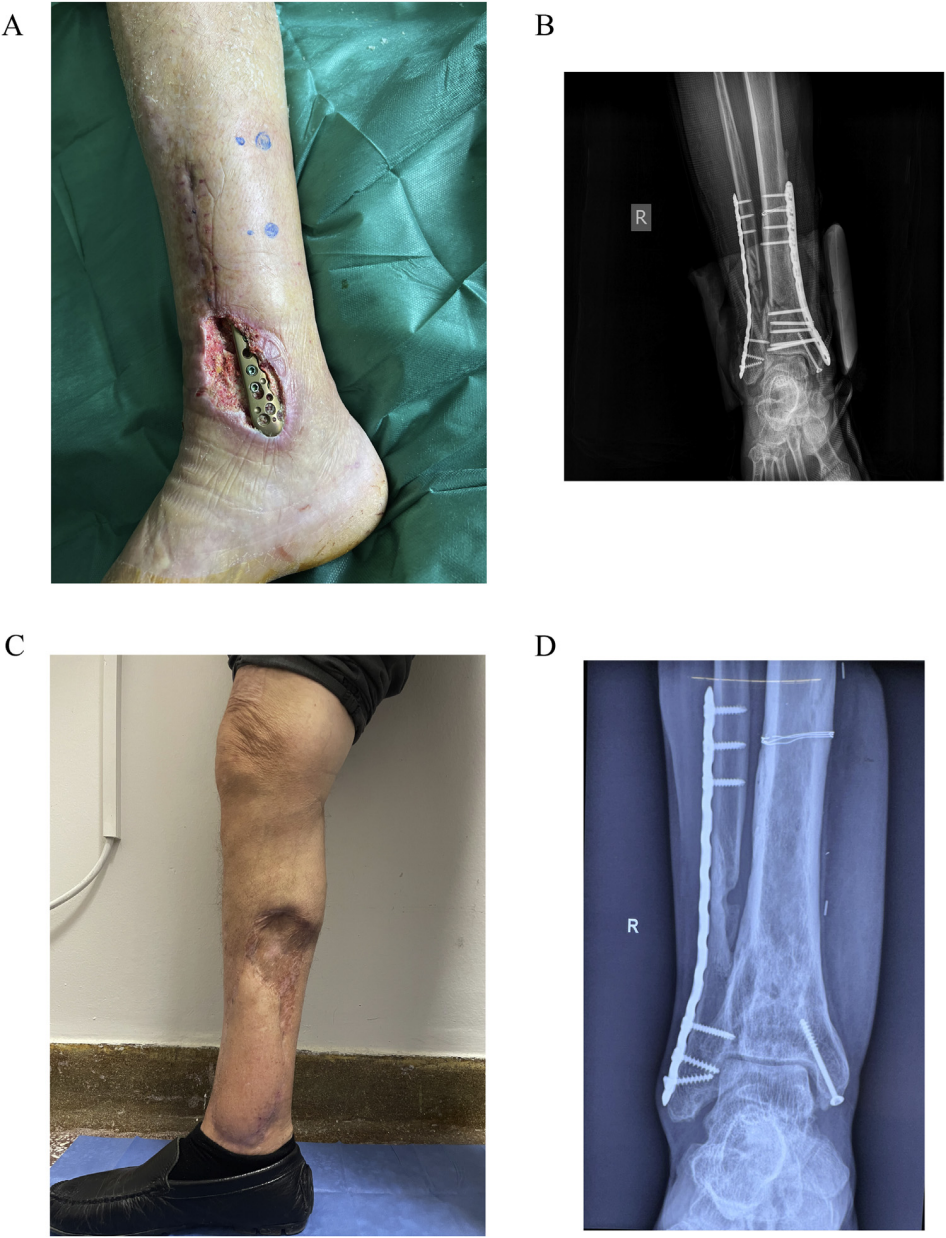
(A) A 72 year-old patient with implant exposure following ORIF of a comminuted distal tibia and fibula fracture. (B) X-ray showing the primary osteosynthesis with plates and screws. (C and D) Postoperative appearance of the reconstructed leg and x-ray showing bony union at 2 years of follow-up.
